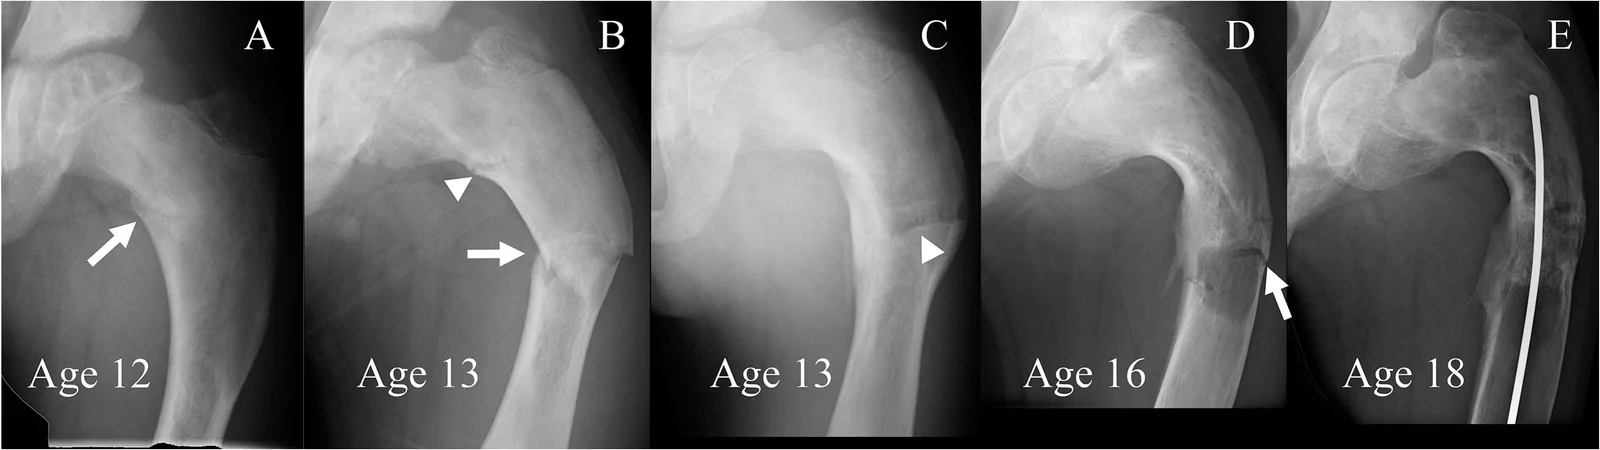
Long-term orthopaedic course of the left femur in Case 1. Serial radiographs show recurrent left femoral pathological fractures, a cortical radiolucency (Looser zone), progressive deformity, and subsequent internal fixation during long-term follow-up. (A) First pathological fracture at age 12. (B) Second pathological fracture at age 13, with a Looser zone in the proximal femur. (C) Persistent Looser zone at age 13 after the second fracture. (D) Third pathological fracture at age 16. (E) Radiograph at age 18 showing the status after repeated pathological fractures, progressive deformity, and internal fixation. Arrows indicate the pathological fracture sites in A, B, and D; arrowheads indicate the Looser zones in B and C.
